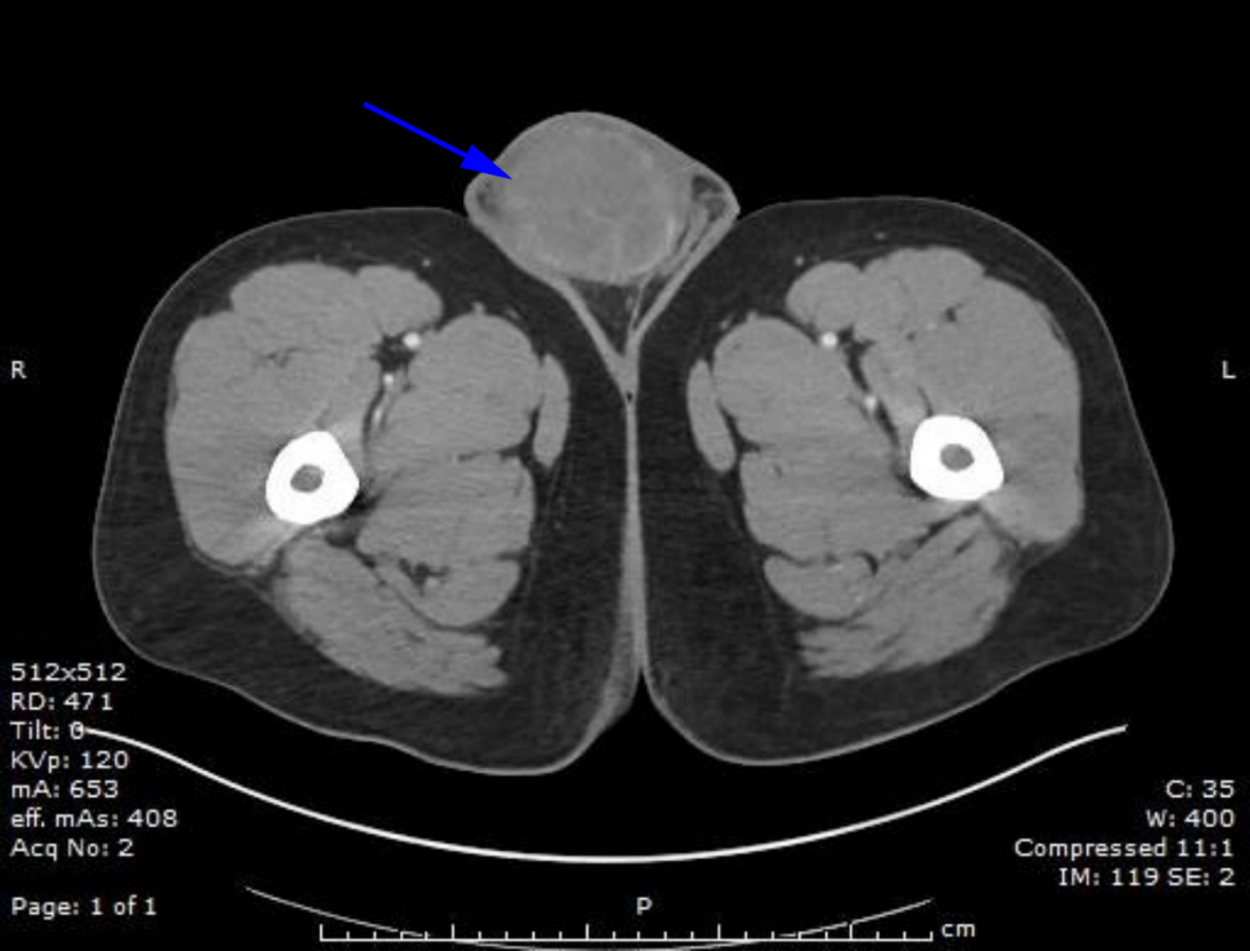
Computed tomography (CT) of the abdomen/pelvis with testicular mass CT of the abdomen and pelvis showing a large right sided testicular mass.
